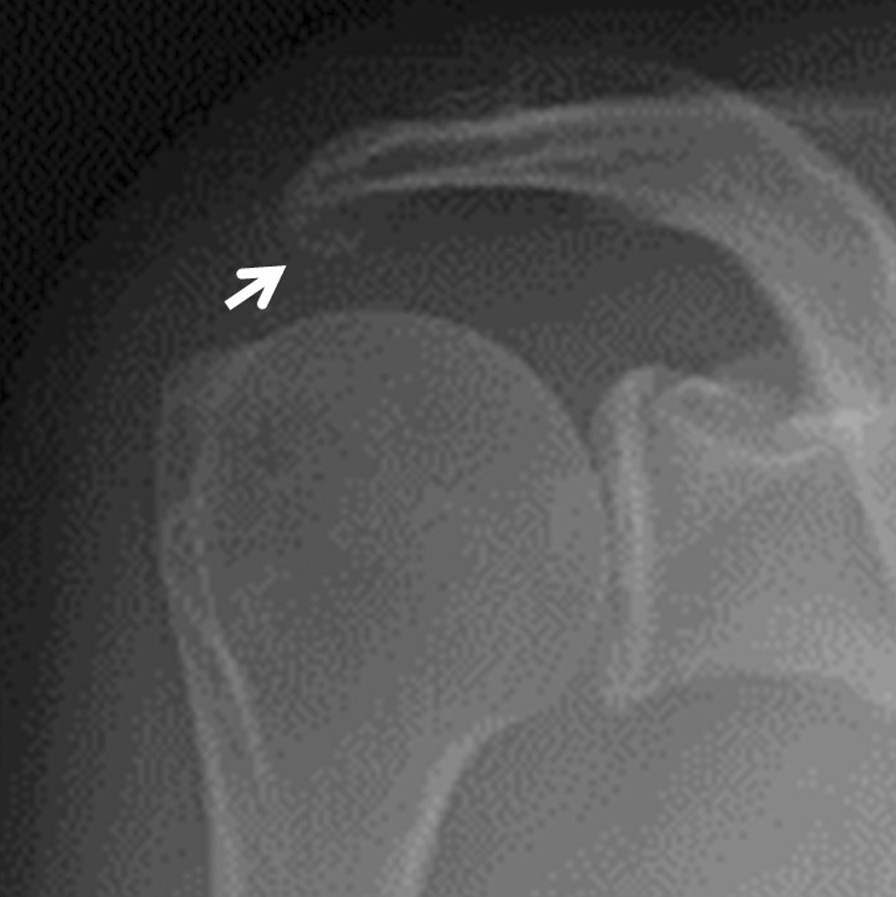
Anteroposterior radiograph of the right shoulder of a 59-year-old Asian woman showing a subacromial spur (arrow)
